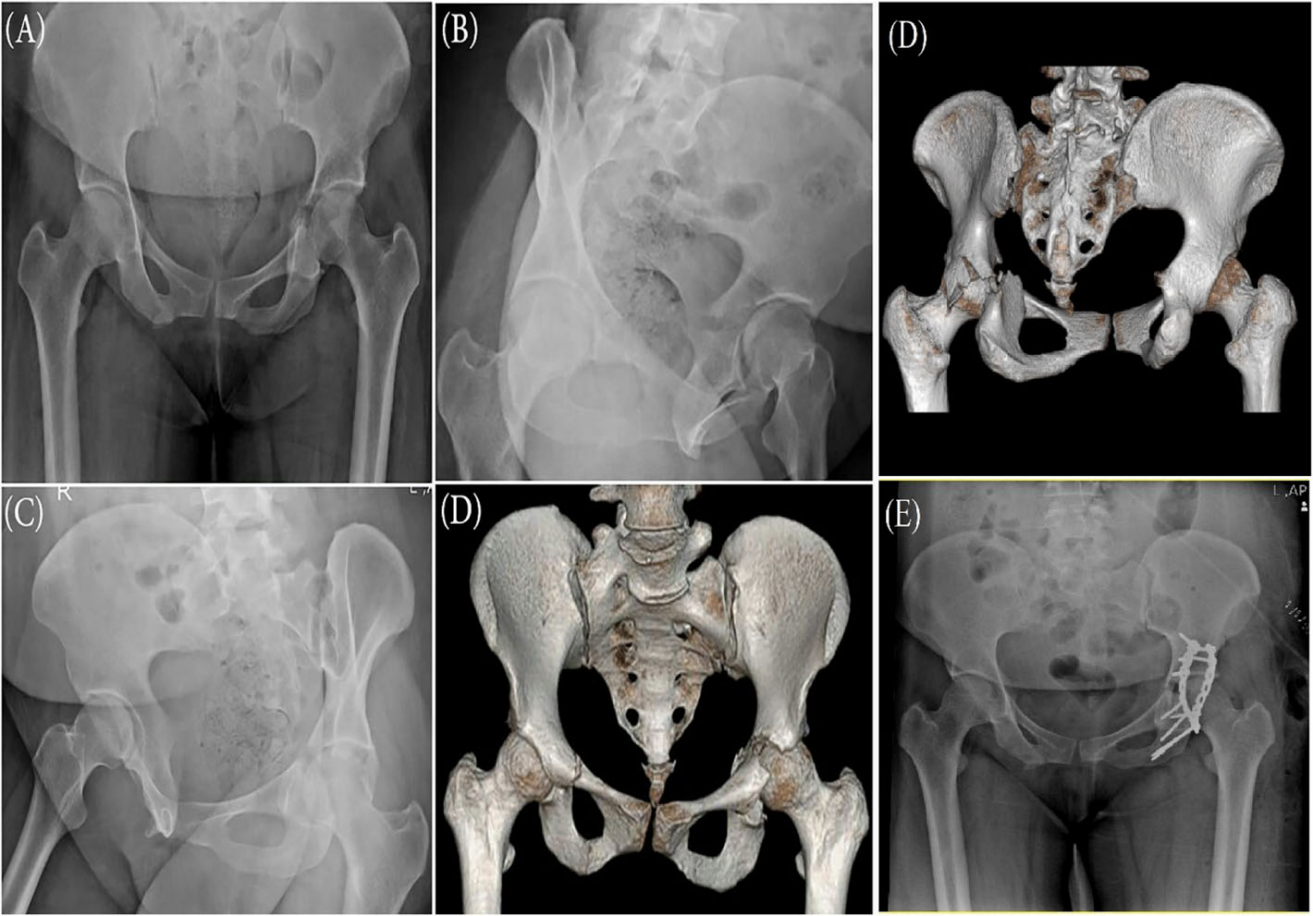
Plain radiographs, a Anteroposterior, b iliac oblique view, c: obturator oblique view. d 3D CT-Scan of a patient with the acetabular fracture. The fracture type was reported as transverse in Ap and Judet view radiographs and after 3d ct scan reported as Transverse & posterior wall fracture that changed the plan and approach of surgery. e postoperative x-ray showing post wall and column fixation with Kocher approach and caused anterior indirect reduction that don’t need surgery
